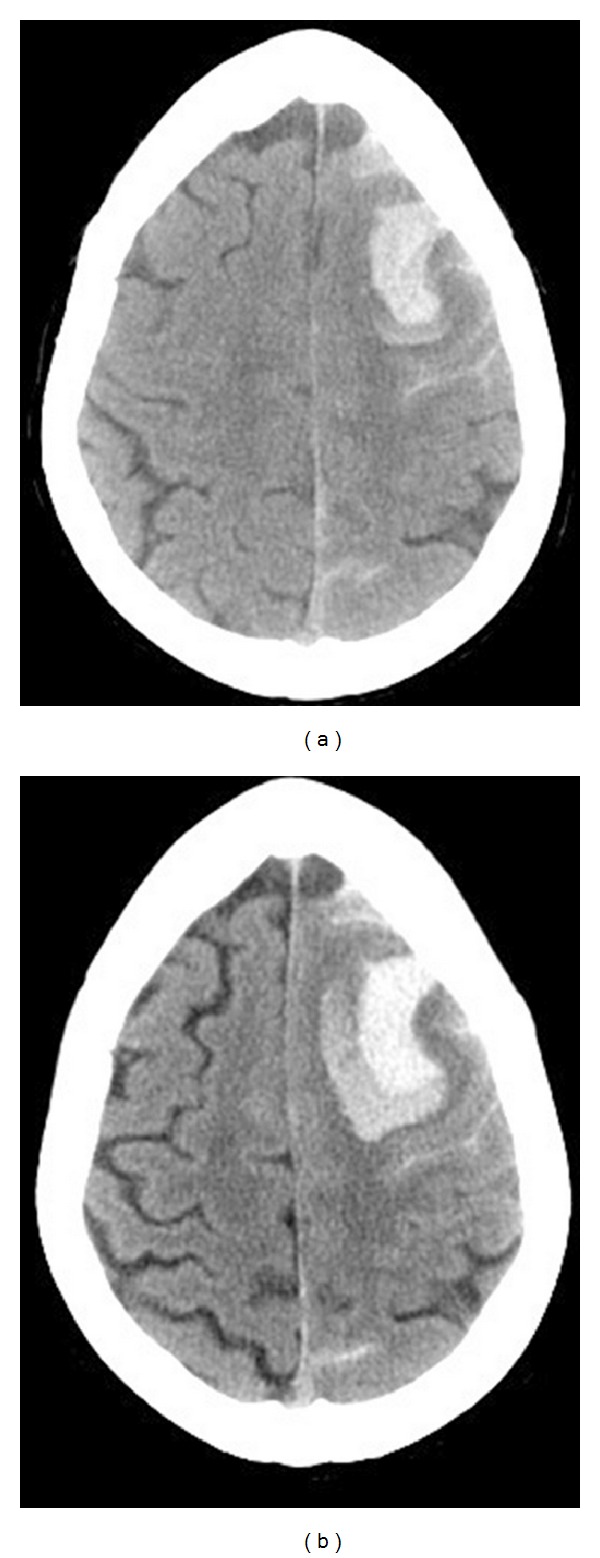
Initial cranial CT without contrast (a) and CT 12 hours later (b). A primary left superior frontal hematoma (3.3 × 1.5 cm) with minimal subarachnoid hemorrhage (a); significant hematoma expansion (4 × 2.5 cm) is evident on the repeat CT (b).
